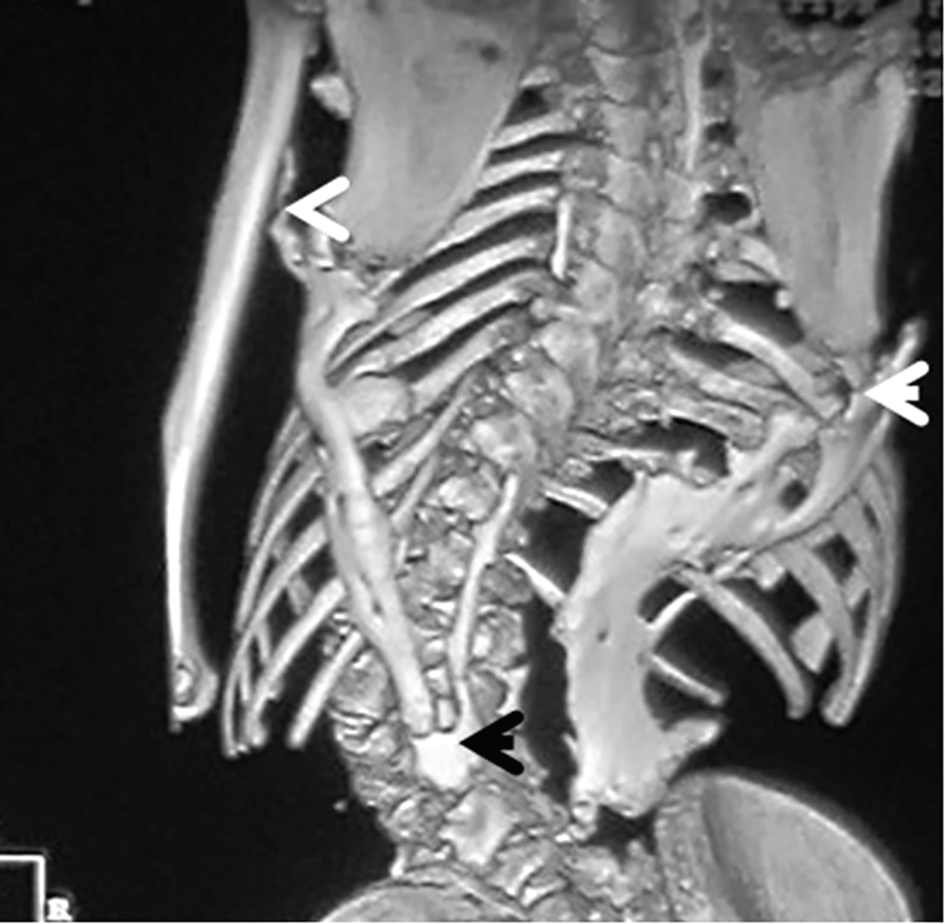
3D reconstruction CT scan in a 16-year-old girl showed diffuse ankylosing ossification of the inferior margins of the scapulae with that of the spine. On the right side, diffuse ankylosing ossification was noted which extended from the inferior margin of the right scapula with a downward manner to cover the posterior aspect of the ribs (7 - 12) until it got ankylosed with the right margin of S1. On the left side, a longitudinal bar of ossification fused superiorly with the inferior margin of the left scapula with downward extension to involve the posterior aspect of the ribs (6 - 12) and bifurcated to two divisions: one extended upward to involve the shaft of the left humerus (arrow head) and the other branch fused with another bar of ossifcation which run in parallel to other bar, which originated from the spinous process of T11 and extended downwards to fuse with the other ossifcation bar at the level of the spinous process of L4 with eventual formation of a dreadful ankylosis (black arrow) (Fig. 3). These progressive episodes of heterotopic ossifications typically lead to dreadful ankylosis of all major joints of the axial and appendicular skeleton, rendering movement impossible, associated with marked elevation in the alkaline phosphatase level (three times greater than normal).
